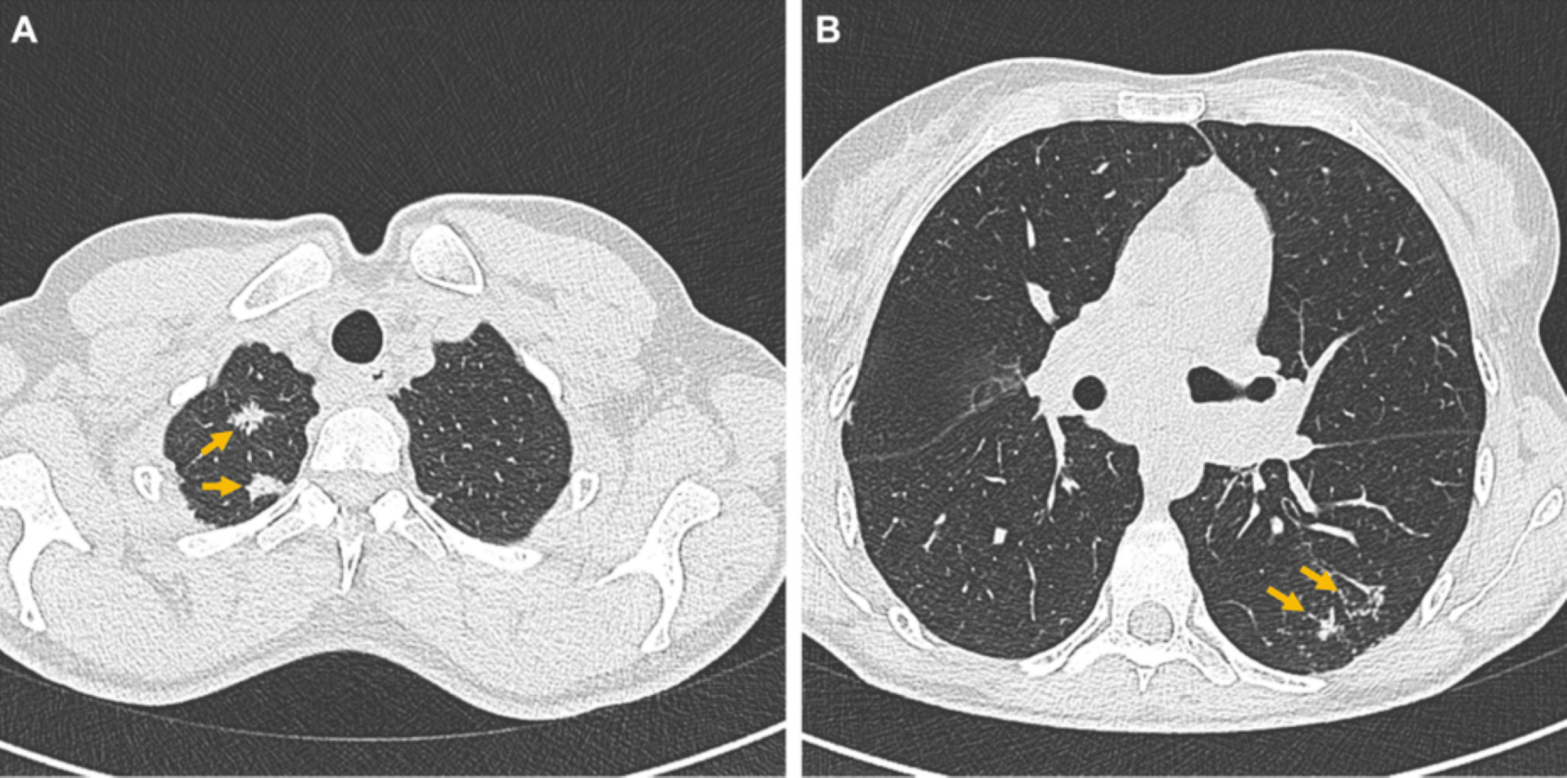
Axial chest CT images showing (A) two spiculated nodules in the right upper lobe and (B) irregular micronodules in the left lower lobe.
